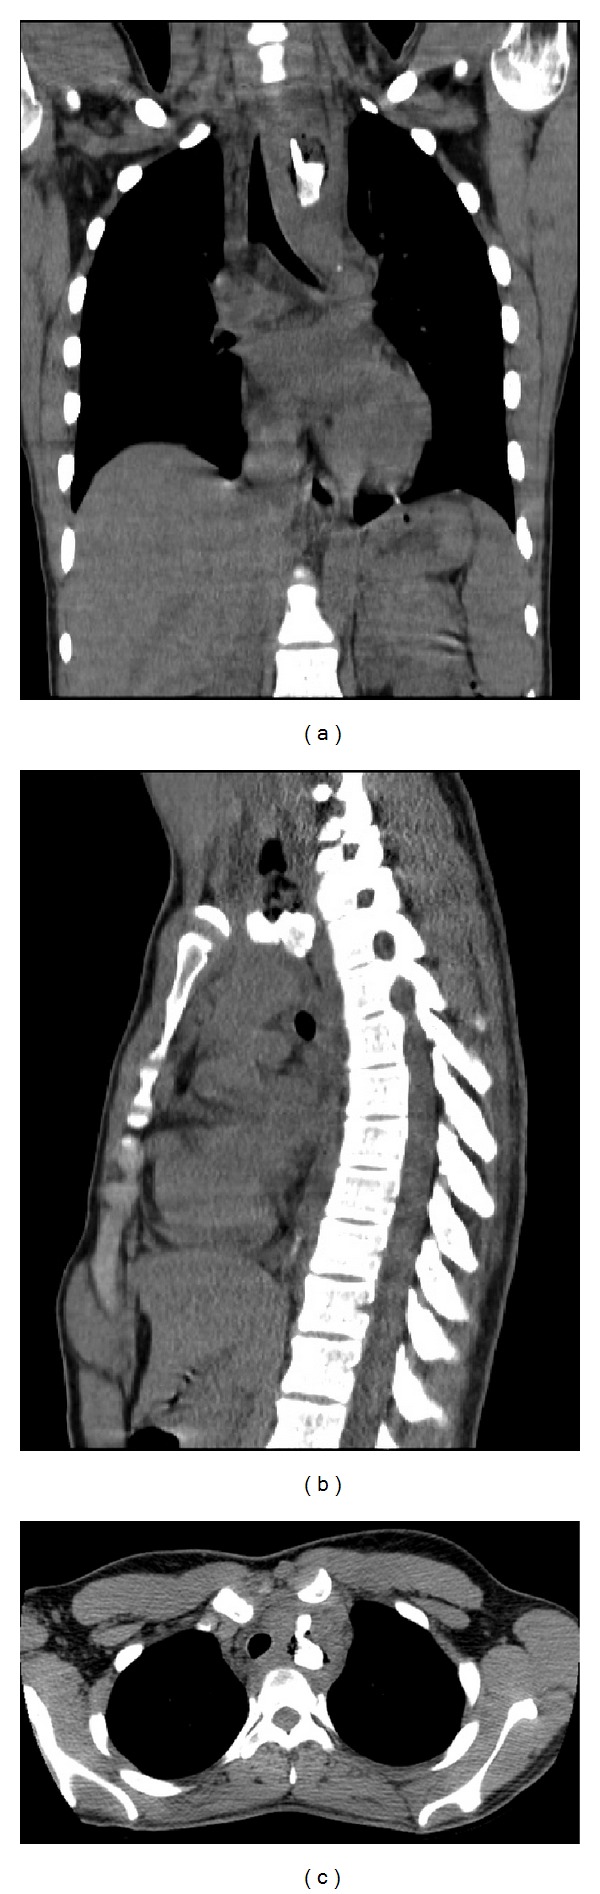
Computed tomography (CT) of the chest without contrast demonstrating a 3.2 cm (cranial/caudal) by 2.5 cm (anterior/posterior) Y-shaped foreign body within the anterior-superior mediastinum in the region of the esophagus. There is gas surrounding the mass. The foreign body has bone density and the gas is in part extraluminal. Edema is present in the circumference of the foreign body and is thought to shift the trachea from the left to the right, with compression of the airway. The soft tissue swelling is also evident surrounding the foreign body and esophagus, reflective of local inflammatory changes and hematoma secondary to the fistula connection between the esophagus and proximal innominate artery. (a) Coronal view. (b) Sagittal view. (c) Axial view.
